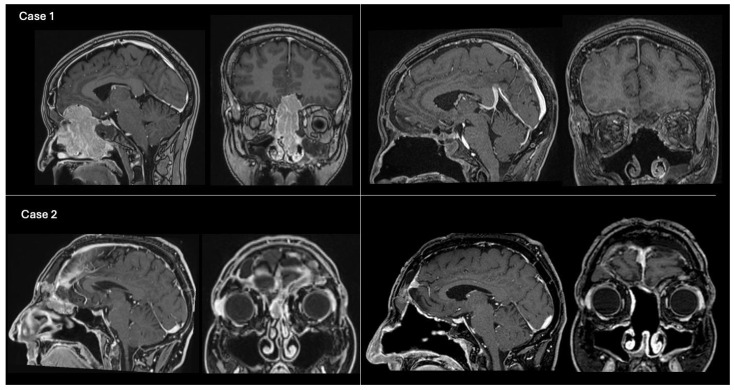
Example cases of preoperative and postoperative T1-weighted contrast-enhanced brain MRI. Case 1 (above): On the left, preoperative MRI on sagittal and coronal views showing an olfactory neuroblastoma (grade C, modified Kadish classification [15]) invading the anterior cranial fossa. The ASB was reconstructed using method 1 (pericranial flap + overlay fascia lata graft). The NSF was not available due to tumor invasion of the nasal septum mucosa. On the right, 1-month postoperative MRI showing gross total resection of the tumor. Case 2 (below): On the left, preoperative MRI on sagittal and coronal views depicting a recurrent ASB atypical meningioma (Grade 2, WHO 2021), previously operated via open approach, with unilateral extension into the right nasal fossa. The ASB was reconstructed using method 2 (pericranial flap + fascia lata graft + NSF). On the right, 2-year postoperative MRI in sagittal and coronal views, demonstrating total tumor removal and intact ASB reconstruction following adjuvant RT.
